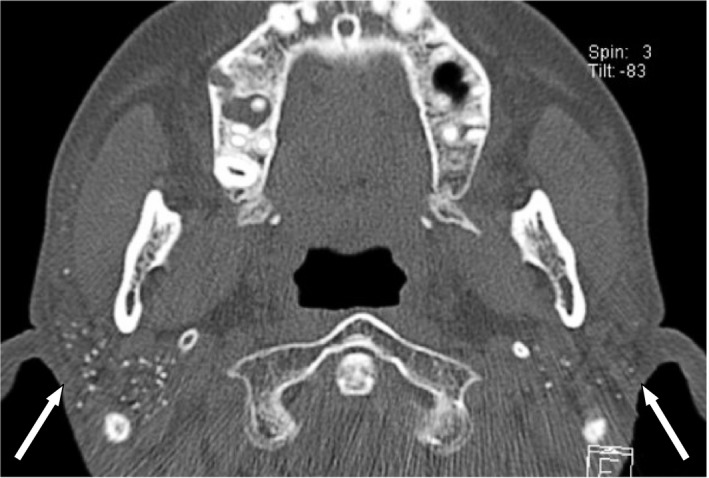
Axial computed tomography shows multiple small calcifications in bilateral parotid glands
